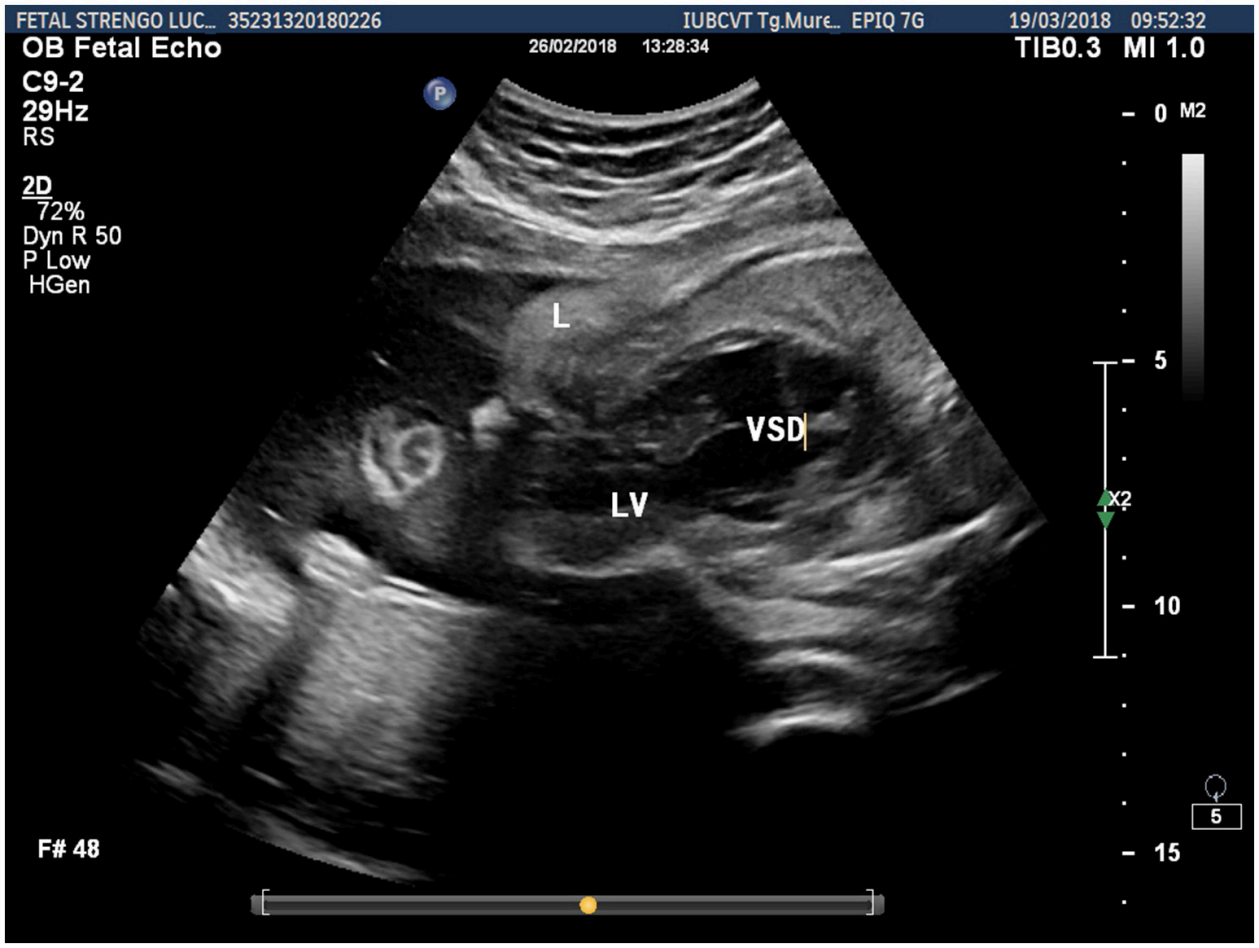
Fetal ultrasonographic image shows extrathoracic left ventricle and extra-abdominal liver (L, liver; LV, left ventricle; VSD, ventricular septal defect).
